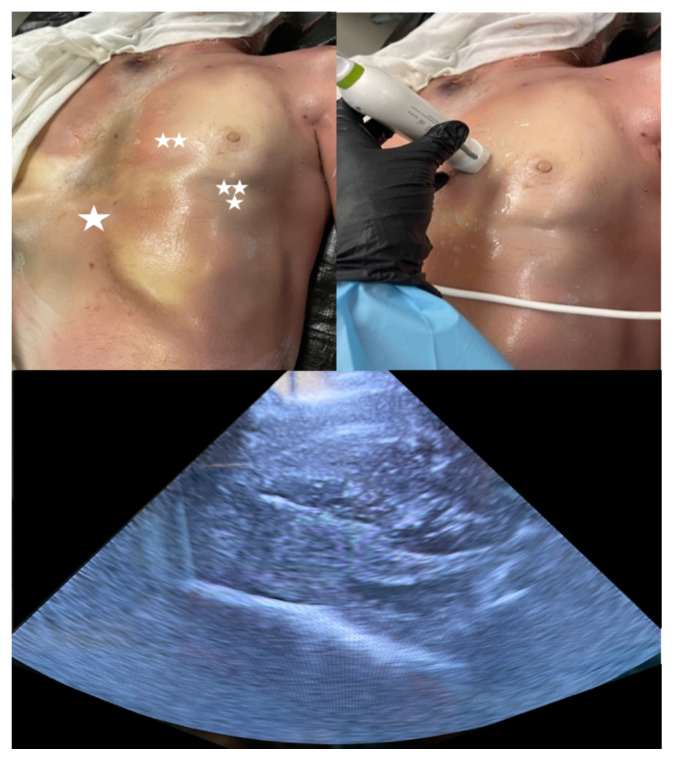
Soft prep cadaver Top left: anatomical location for subxiphoid (one star), parasternal (two stars) and apical (three stars) approaches. Top right: using ultrasound to demonstrate parasternal view. Bottom: parasternal view prior to creation of effusion
